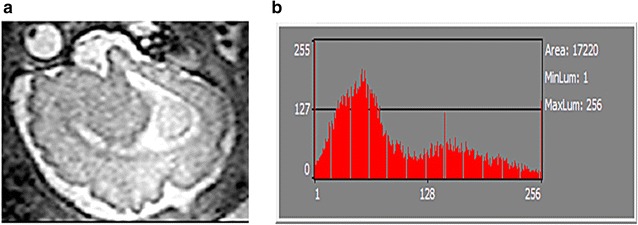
Histogram representation of fetal brain. a MRI of fetal brain. b Histogram of the whole image
